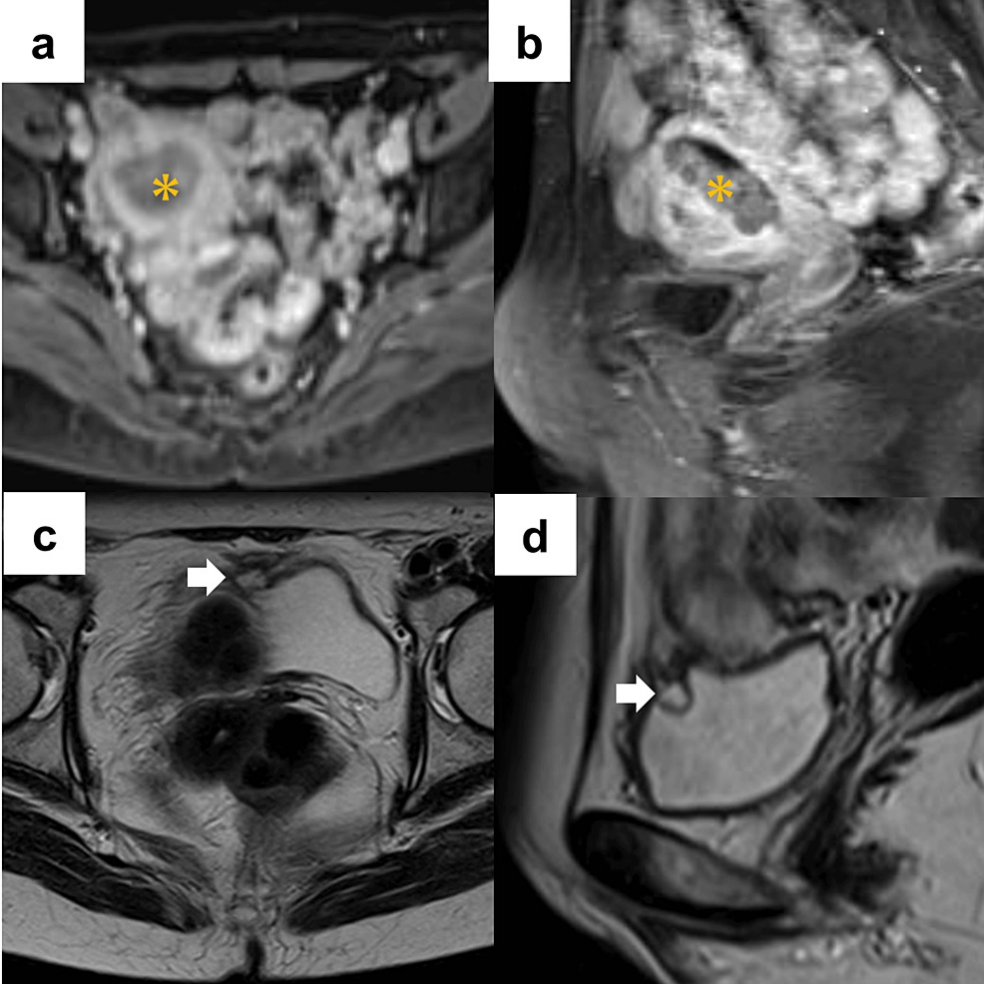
Magnetic resonance imaging of endometrial cancer and bladder cystic lesion a and b: Gadolinium-enhanced magnetic resonance imaging revealed endometrial cancer in stage ⅠA, according to the International Federation of Gynecology and Obstetrics (FIGO) staging classification (*) (a: axial section and b: coronal section). c and d: Magnetic resonance imaging (T2-weighted) showed a 2-cm cystic lesion at the bladder dome (arrow) (c: axial section and d: coronal section).
